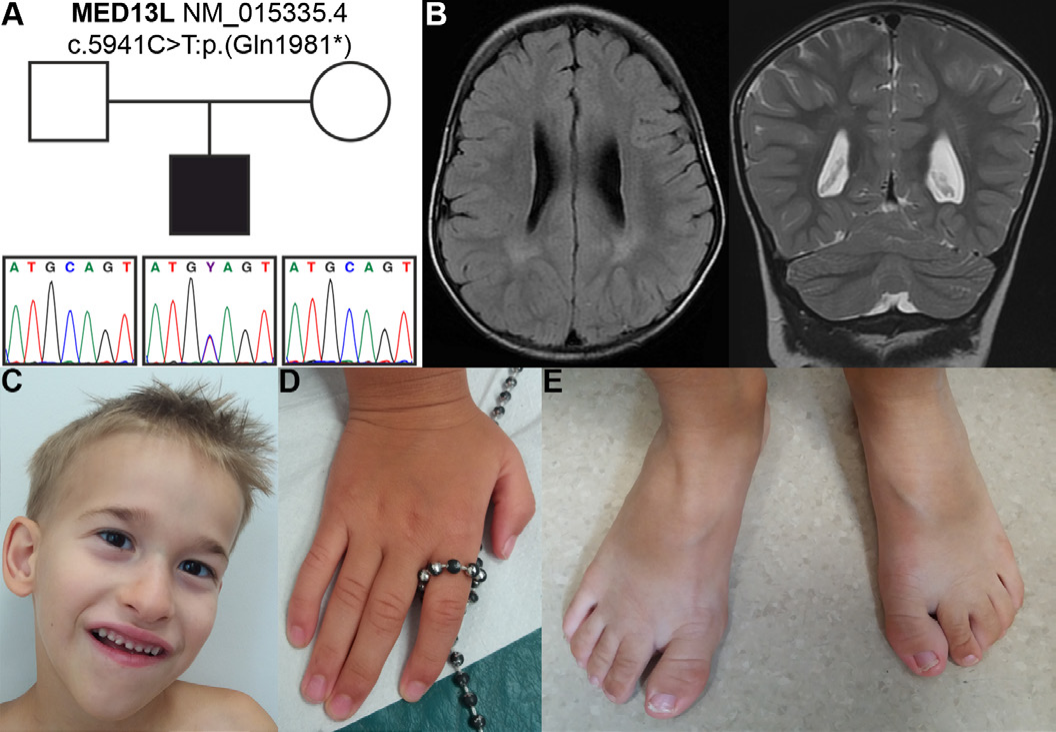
(A) Sanger sequencing confirmed the nonsense variant c.5941C>T in the patient. (B) Axial and coronal brain MRI showing terminal zones of unfinished myelination posteriorly and asymmetric ventricles as well as the Virchow-Robin spaces in the same location. (C) Facial features, (D) hands and (E) feet anomalies in patient at 7 years of age.
